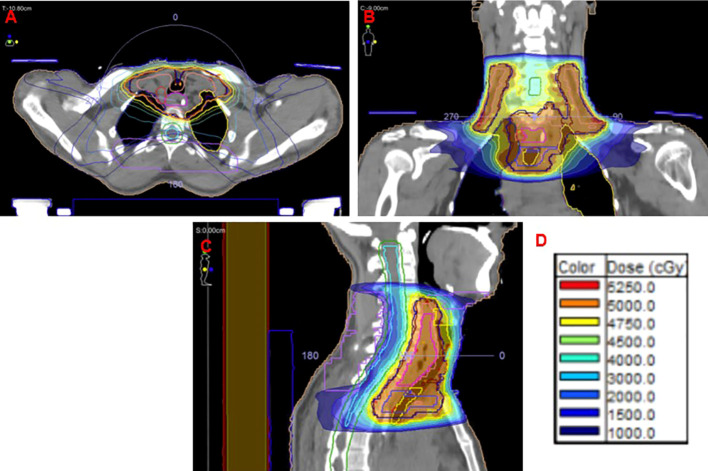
Radiation therapy treatment plan. (A) Axial, (B) coronal, nd (C) sagittal views from the treatment planning CT showing target volumes isodose distributions. (D) Color scale depicting radiation dose levels in centigray (cGy) corresponding to Panels (A-C).
